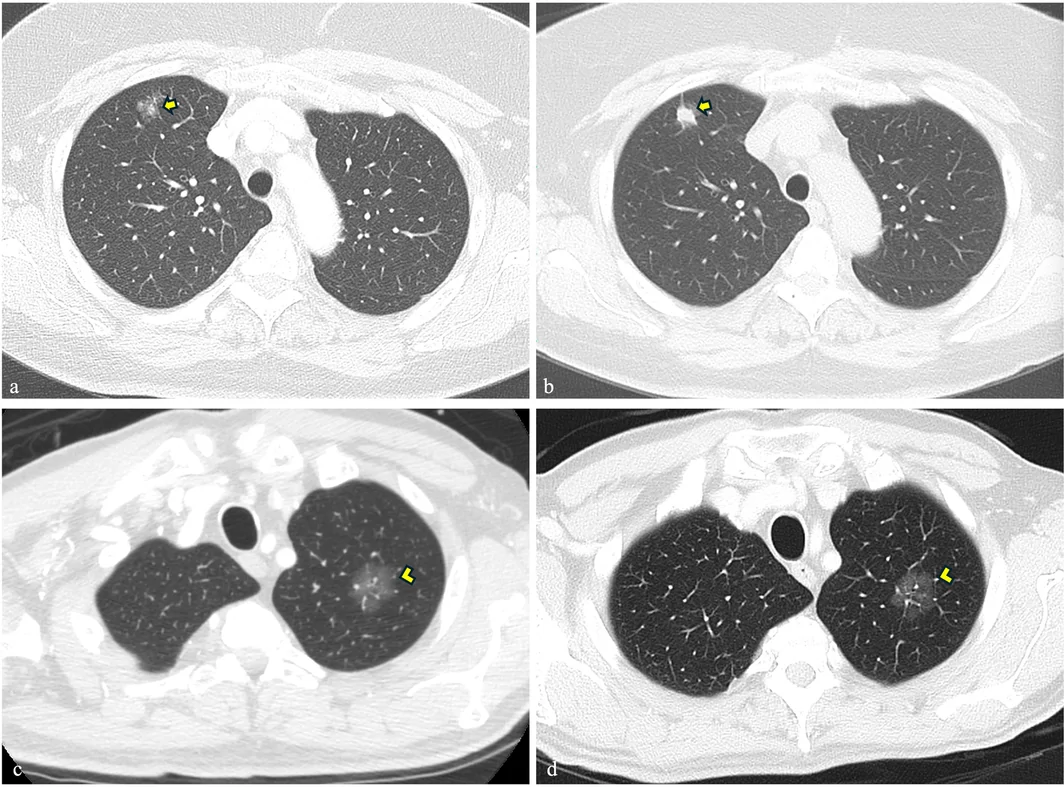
(a) Axial computed tomography of a 65‐year‐old woman with a ground glass nodule in the right upper lobe (yellow arrow) that at follow‐up 2 years later underwent consolidation (yellow arrow in b). (c) Axial computed tomography of a 73‐year‐old man with a ground glass nodule in the left upper lobe (yellow arrowhead) that at follow‐up 1 year later remained stable without any sign of consolidation (yellow arrowhead in d).
